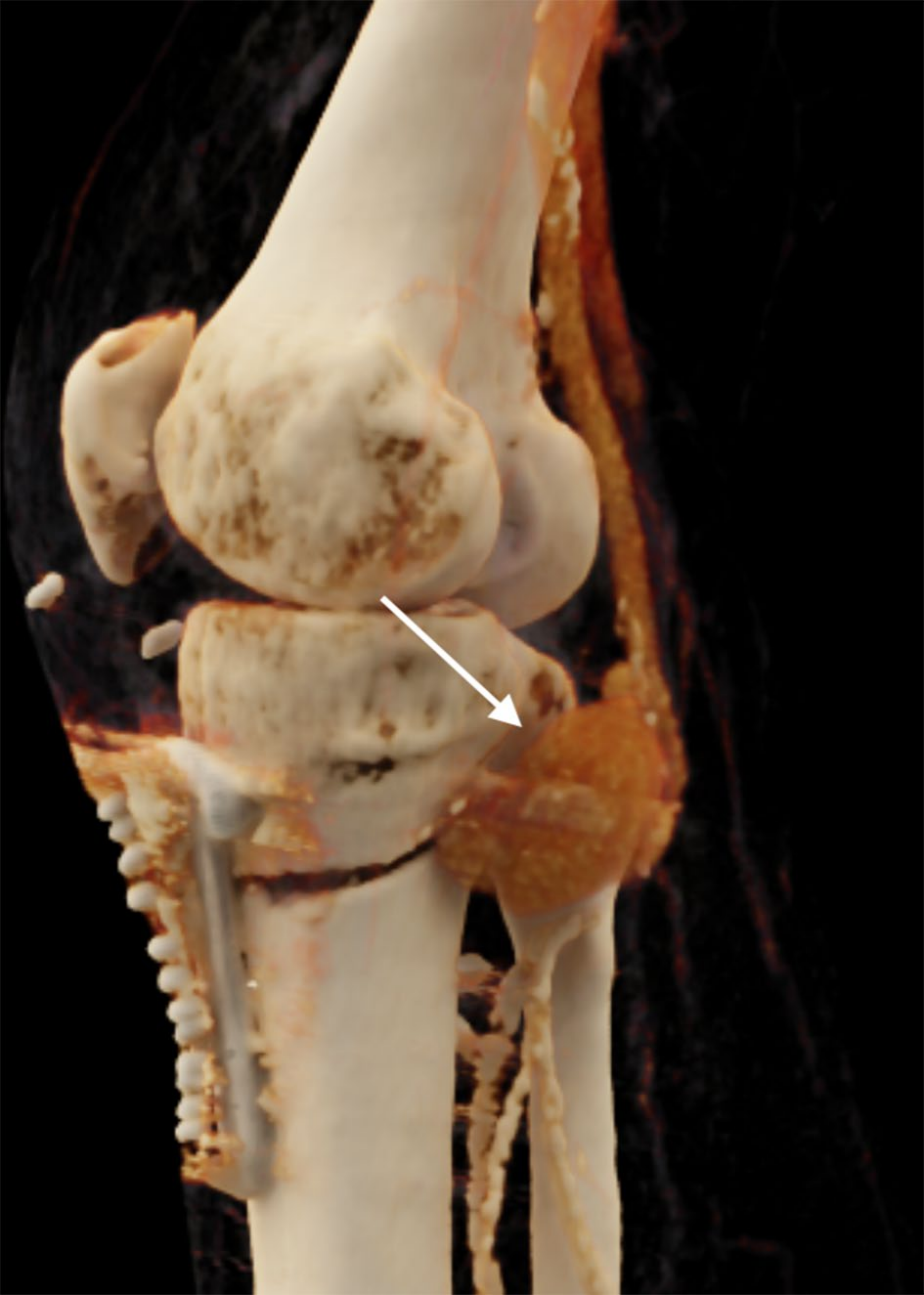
3D reconstruction of postoperative situs after high tibial realignment osteotomy from contrast-enhanced computed tomography. Shown is the course of the osteotomy and the spatial proximity to the popliteal artery, which shows contained rupture (arrow)
